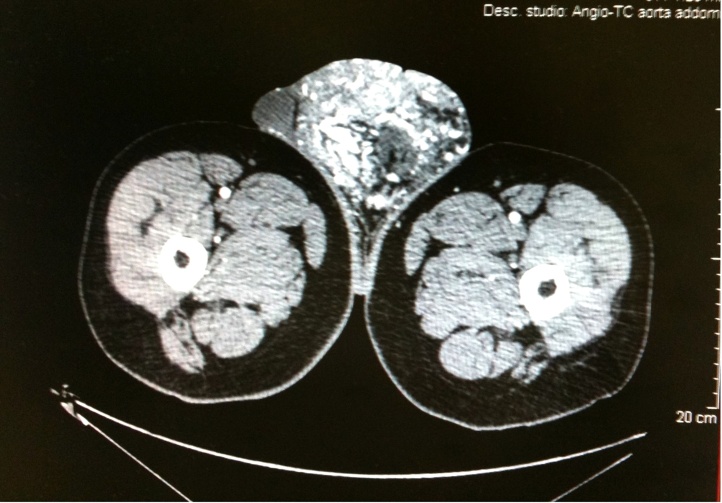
Angio-CT MIP of the lower abdomen and the cranial portion of inferior limbs: See the presence of a raw agglomerate of enlarged blood vessels located in the left-side of the scrotum and contralateral dislocation of the right testicle.
